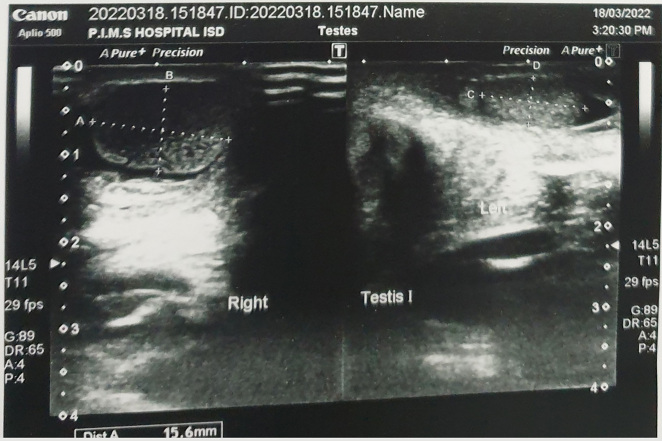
Doppler ultrasound and heterogeneous changes in the right testis.
